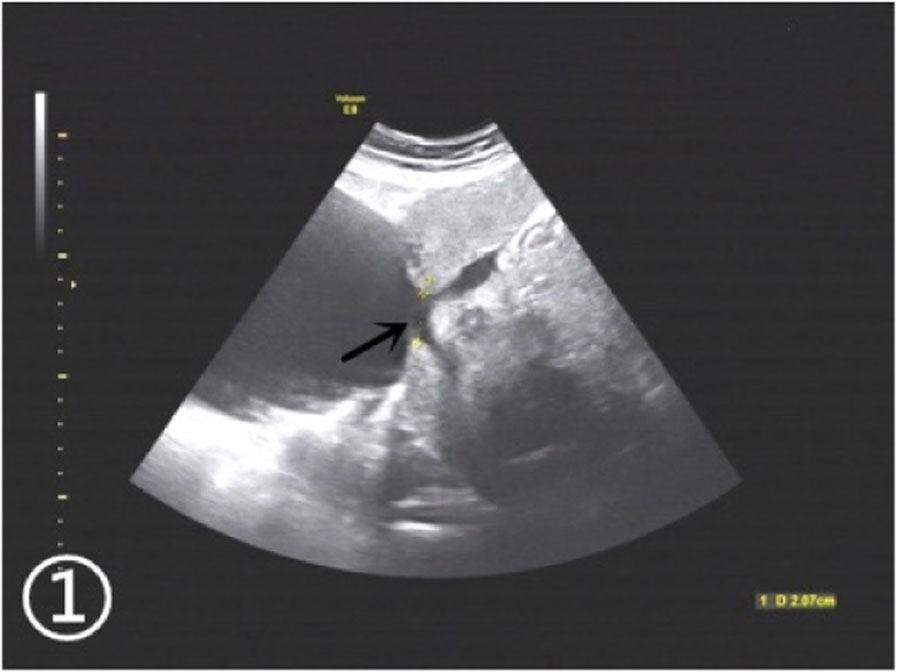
Ultrasound scan image: myometrial defect at the right uterine horn and hypoechoic liquid dark area connected to the uterine cavity
